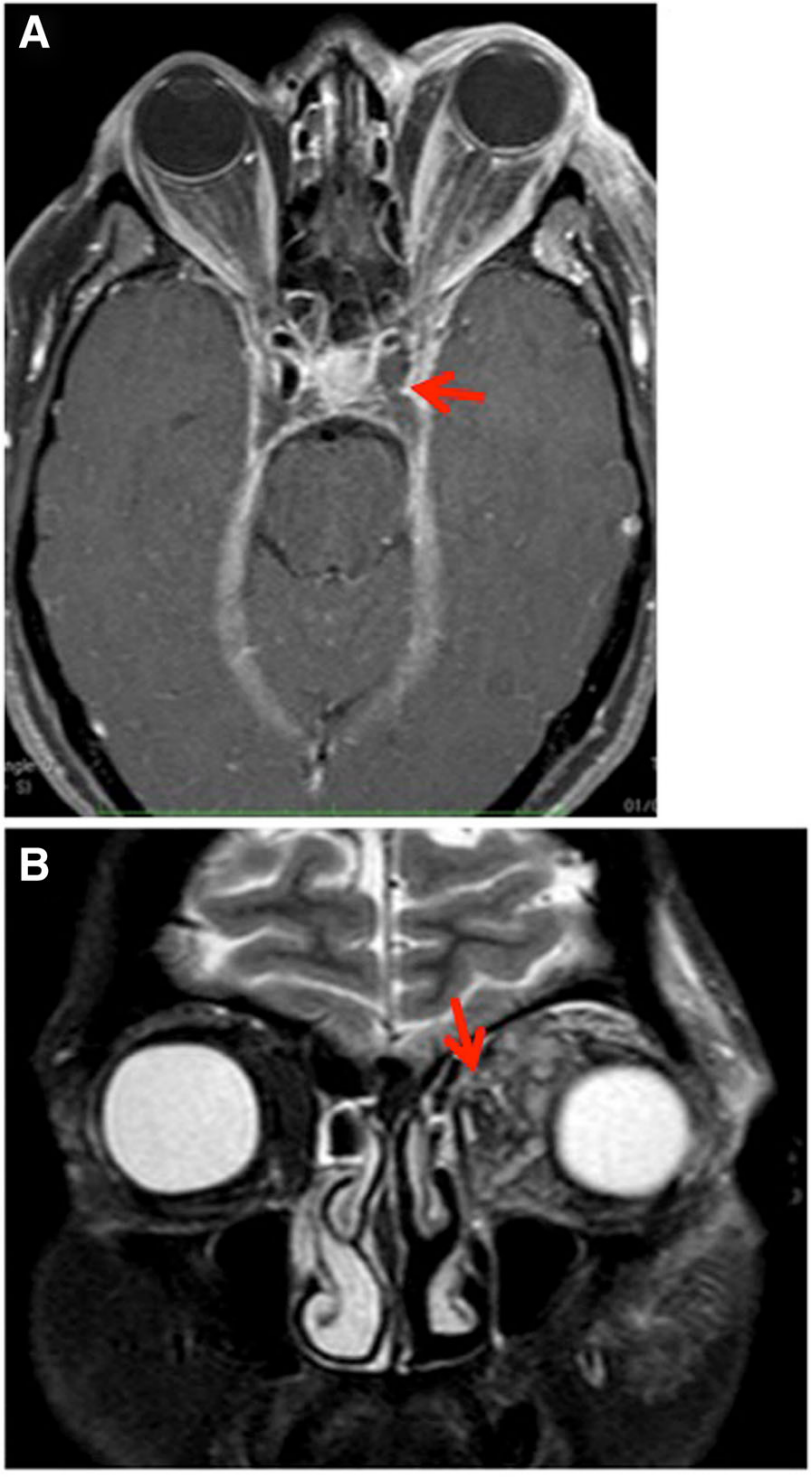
Contrast-enhanced magnetic resonance imaging. a A non-fat density filling defect in the *left side* of the cavernous sinus. b Coronal plane, periorbital swelling (*arrow*)
